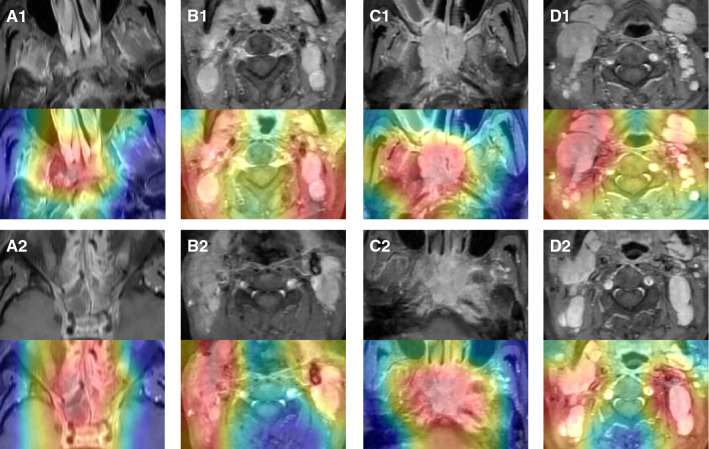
CAM examples for various MR images using InceptionResNetV2. A1 and A2 Tumor T2 MRIs of stage III and IVa, B1 and B2 lymph node T2 MRI of stage III and IVa, C1 and C2 Tumor T1 MRI of stage III and IVa, D1 and D2: lymph node T1 MRI of stage III and IVa
